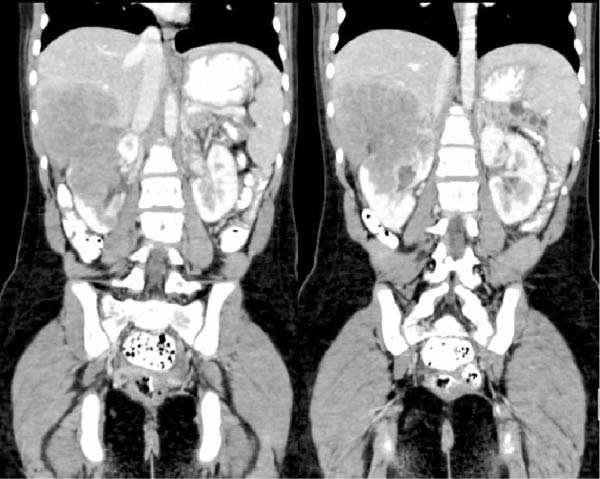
Abdominal CT of the patient reveals a heterogeneous mass in the right kidney and liver.
